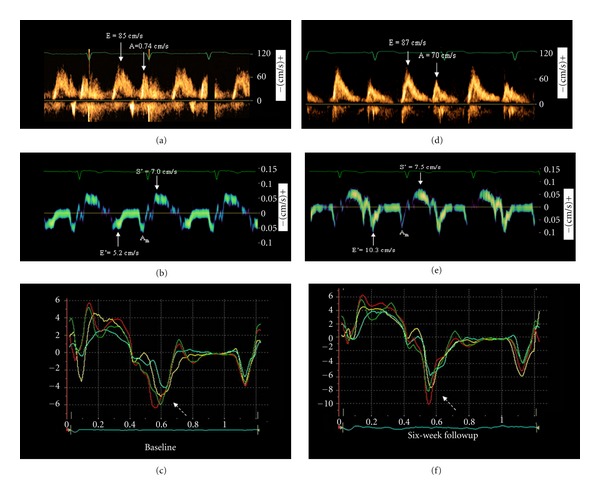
Left ventricular diastolic function at baseline and at the 6-week followup. Left column: Baseline examination. Doppler pattern of transmitral flow (a), Tissue Doppler pattern of left ventricular (LV) motion recorded at the junction with the septal mitral annulus (b), and color Tissue Doppler velocities of multiple LV myocardial segments ((c): yellow, basal septum; turquoise, middle septum; red, basal lateral; green, middle lateral). Severe reduction of peak early diastolic myocardial velocity (E_m_) and considerable early diastolic dyssynchrony between segments (dotted arrow) were present. Right column: corresponding images obtained after 6 weeks of therapy with bromocriptine. Transmitral Doppler pattern was substantially unchanged (d), whereas a considerable increase in E′ (e) and a reduction in early diastolic dyssynchrony ((f), dotted arrow) were observed. Also note the different velocity scales between (c) and (f), and the increase in early diastolic velocities of all myocardial segments at 6-week followup compared to baseline. A = peak late diastolic transmitral flow; A′ = peak late diastolic myocardial velocity; E = peak early diastolic transmitral flow; S′ = peak systolic myocardial velocity.
